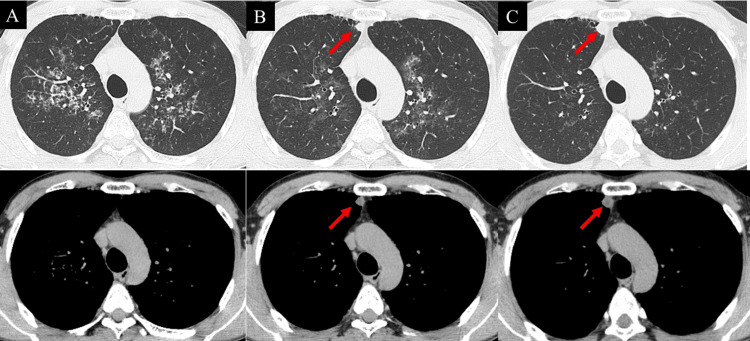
Chest computed tomography features before the appearance of a nodule (A), when a nodule was newly found in the right upper lobe (B), and six months after the detection of the nodule (C). The arrow on each figure indicates the nodule.
